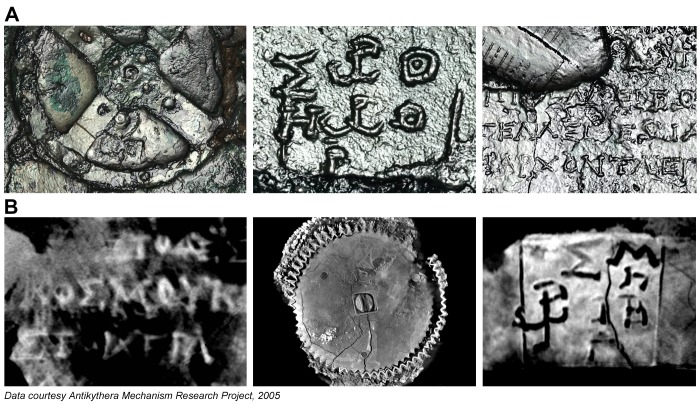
Examples of data gathered in 2005 on the Antikythera Mechanism. (A) Polynomial Texture Mapping (PTM) with specular enhancement [2]. (B) High-resolution Microfocus X-ray Computed Tomography (X-ray CT) [3].
